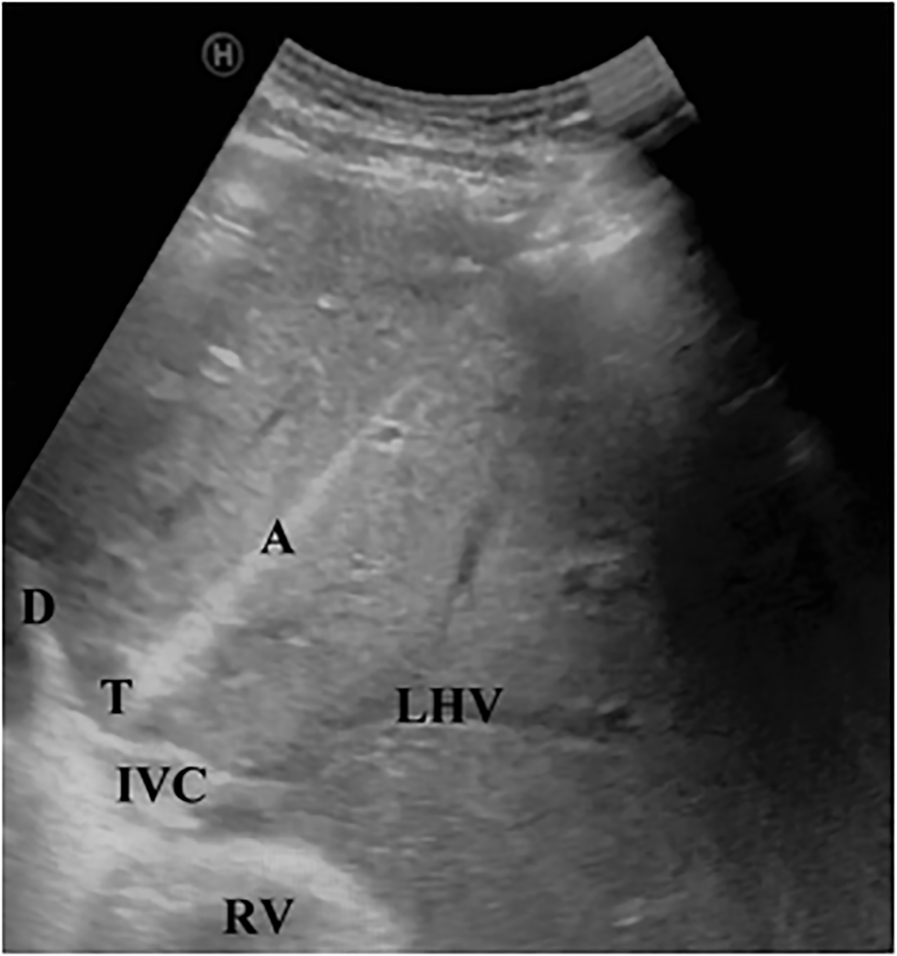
Microwave ablation needle insertion in the hepatocellular carcinoma liver nodule under ultrasound guidance. *A* antenna microwave, *D* diaphragm, *IVC* inferior vena cava, *LHV* left hepatic vein, *RV* right ventricle, *T* cancer
